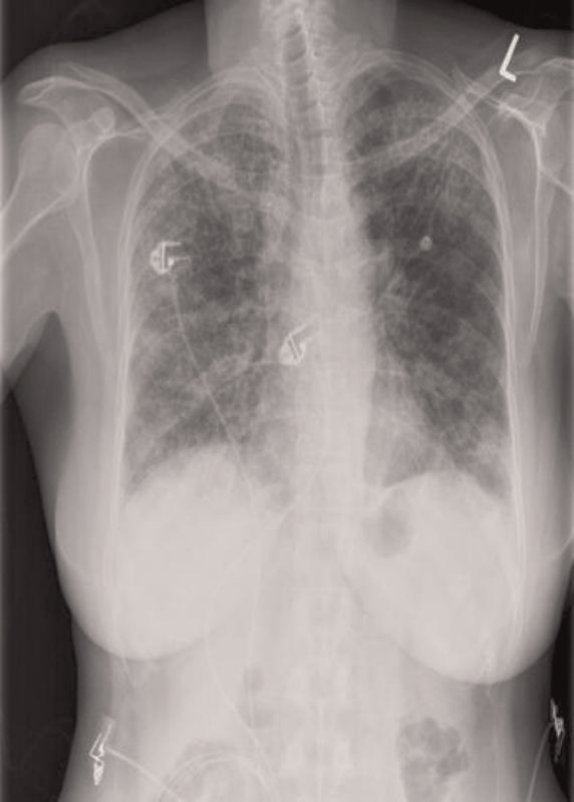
Chest radiograph showing diffuse, patchy bilateral interstitial infiltrates.
